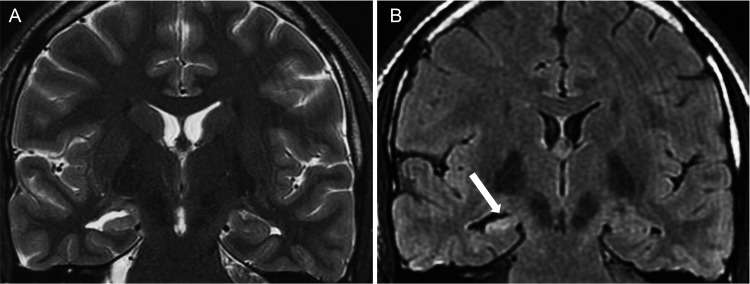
Hippocampal sclerosis in a 31-year-old man with refractory right mesial temporal sclerosis. A) coronal T2-weighted image and B) coronal FLAIR images, both perpendicular to the long axis of the hippocampus. These images show a hyperintense signal, the loss of digitations and atrophy in the right hippocampal head (white arrow).
